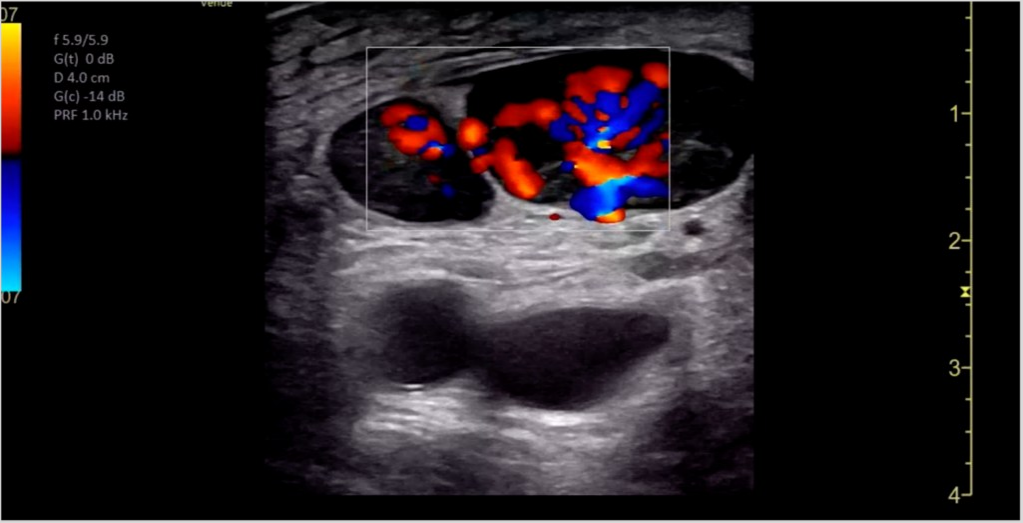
Point of care ultrasound (POCUS) with color Doppler. Diffuse internal vascularity is shown, previously described as “fire pattern.”
